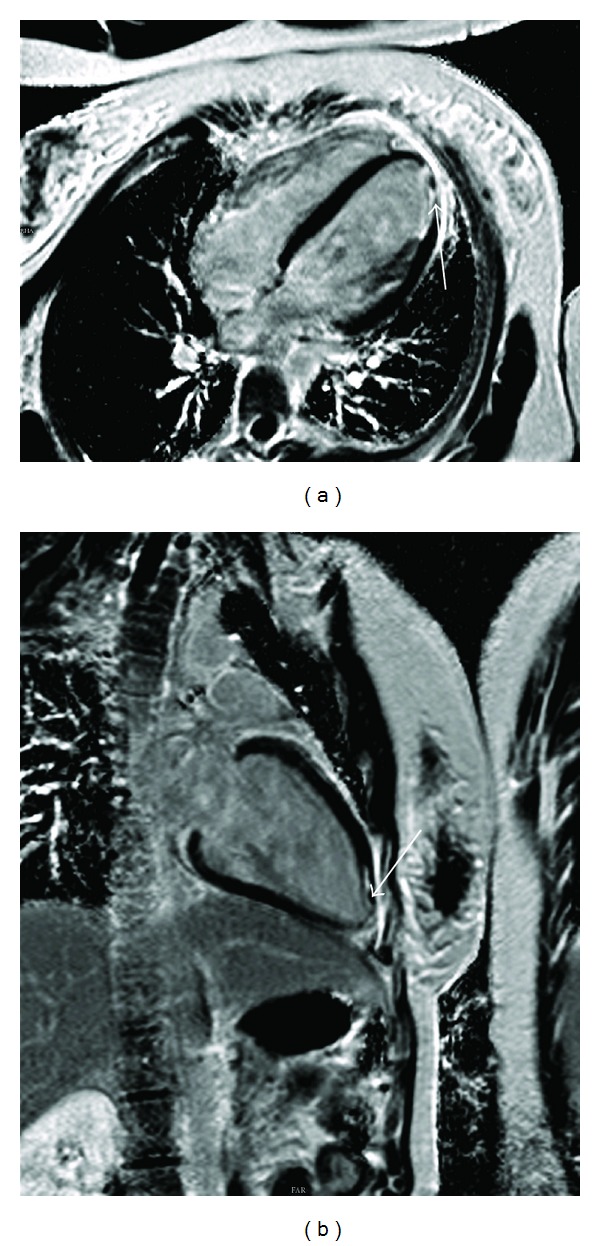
After ablation, a cardiac MRI study was performed. MRI images showed a slightly dilated left ventricle. End diastolic LV volume was 213 mL. End systolic LV volume was 112 mL. Stroke volume was 101 mL, LVEF was 47% and IVSDd was 11 mm. Gadolinium was used to evaluate myocardial perfusion. At the apicolateral side of the LV, a small area with transmural late enhancement was identified.
